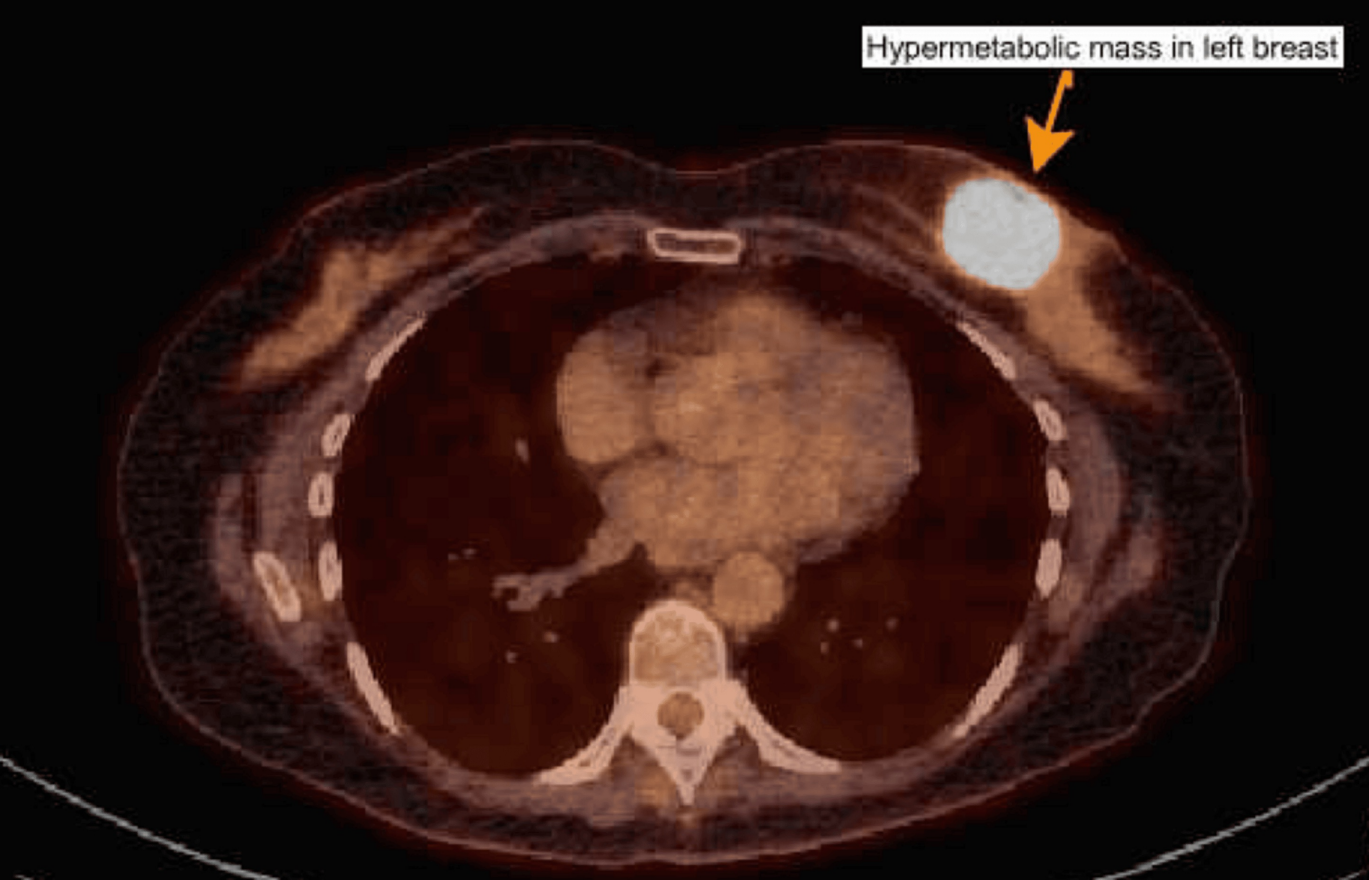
PET-CT showing a hypermetabolic mass in the left breast PET-CT: positron emission tomography/computed tomography
